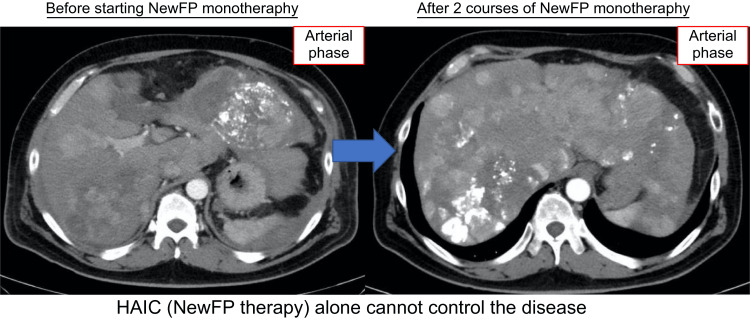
After two courses of the New FP therapy, contrast-enhanced CT evaluation revealed an increase in target lesions, the appearance of new intrahepatic lesions, and an upward trend in tumor markers. HAIC: hepatic arterial infusion chemotherapy
